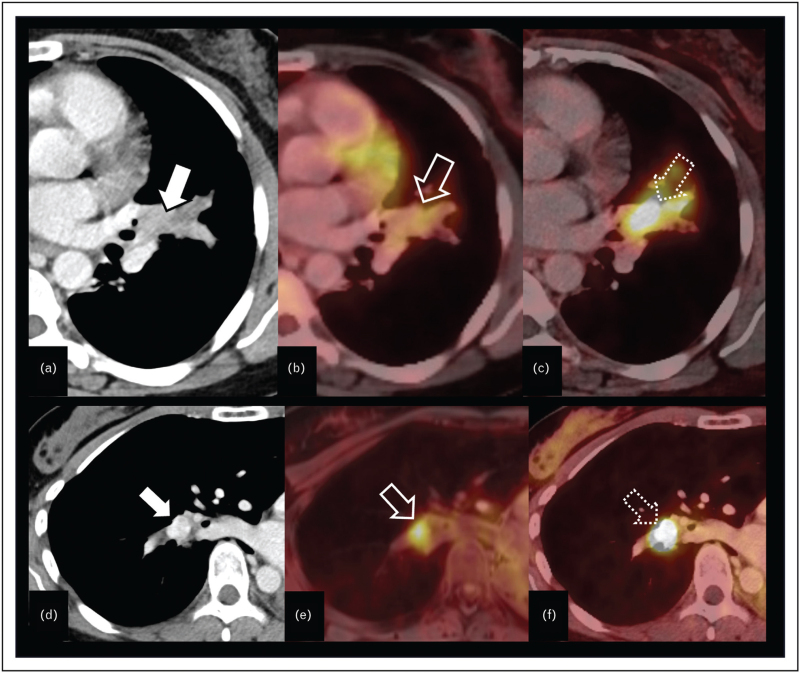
Transverse CT image reconstruction shows an endobronchial tumor (a) (white arrow) on the left, which does not show any FDG-uptake (open arrow) on ^18^FDG-PET/CT scan (b) but somatostatin expression (dashed arrow), and therefore positivity on DOTANOC-PET CT scan (c), highly suggestive of a typical carcinoid. Transverse CT image reconstruction shows an endobronchial tumor (d) (white arrow) on the right, which shows an FDG-uptake (open arrow) on ^18^FDG-PET/CT scan (e) and somatostatin expression (dashed arrow) and therefore positivity on DOTANOC-PET CT scan (f), highly suggestive of an atypical carcinoid.
